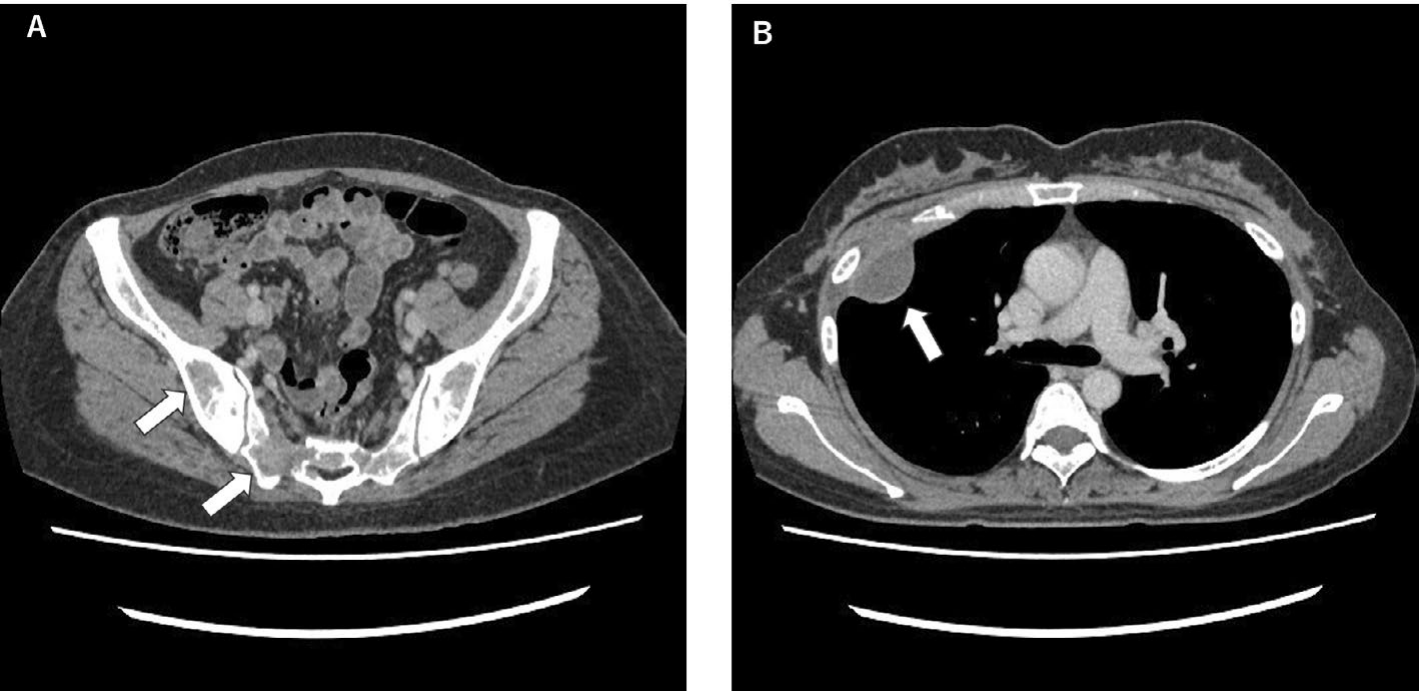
Abdominal and Chest Computerized Tomography Imagings (Case 1). An abdominal CT imaging of Case 1 showed multiple osteolytic lesions involving pelvic bones (Panel A, indicated by arrows). A Chest CT imaging showed a soft‐tissue mass developed over the right chest wall (Panel B, indicated by an arrow).
